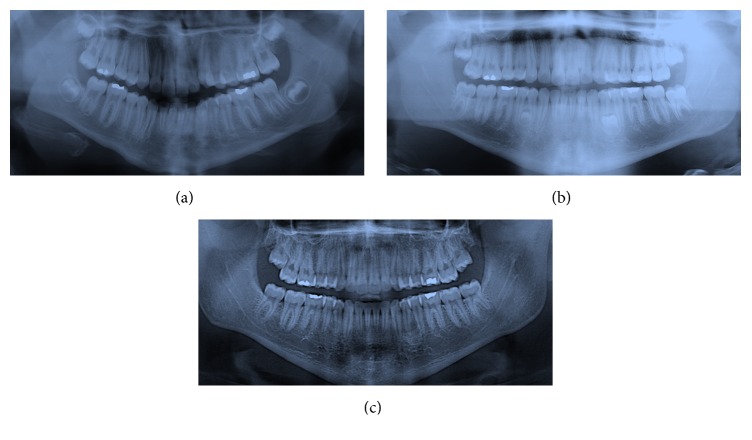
Case 4 radiography: before treatment 2011 (a), after treatment, ST in regions 3.4–3.5 and 4.4–4.5, 2014 (b), and after extraction of ST 2014 (c).
